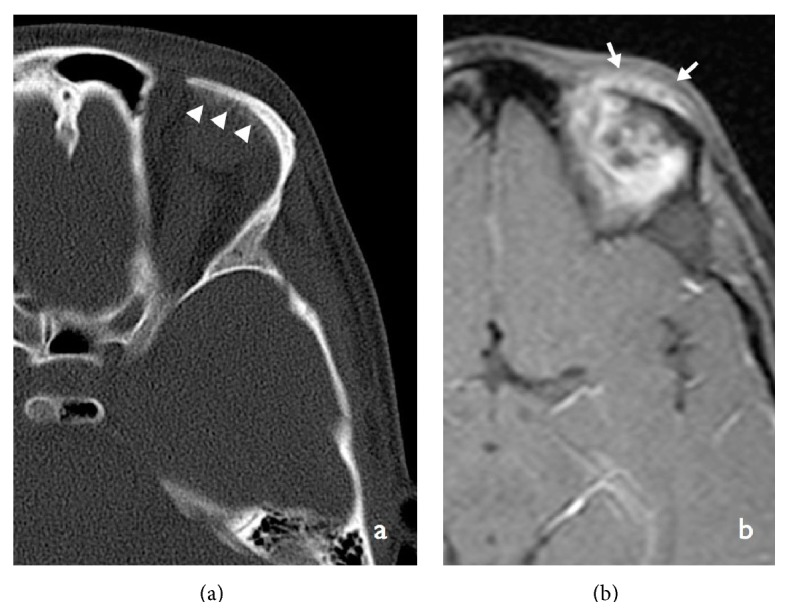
Axial noncontrast enhanced CT section in bone window projection (a) showing periosteal reaction exerted by the lesion on the superior orbital ridge seen as amorphous density. Contrast-enhanced sagittal T1-weighted MRI image (b) showing an amorphous area of enhancement of the lesion.
